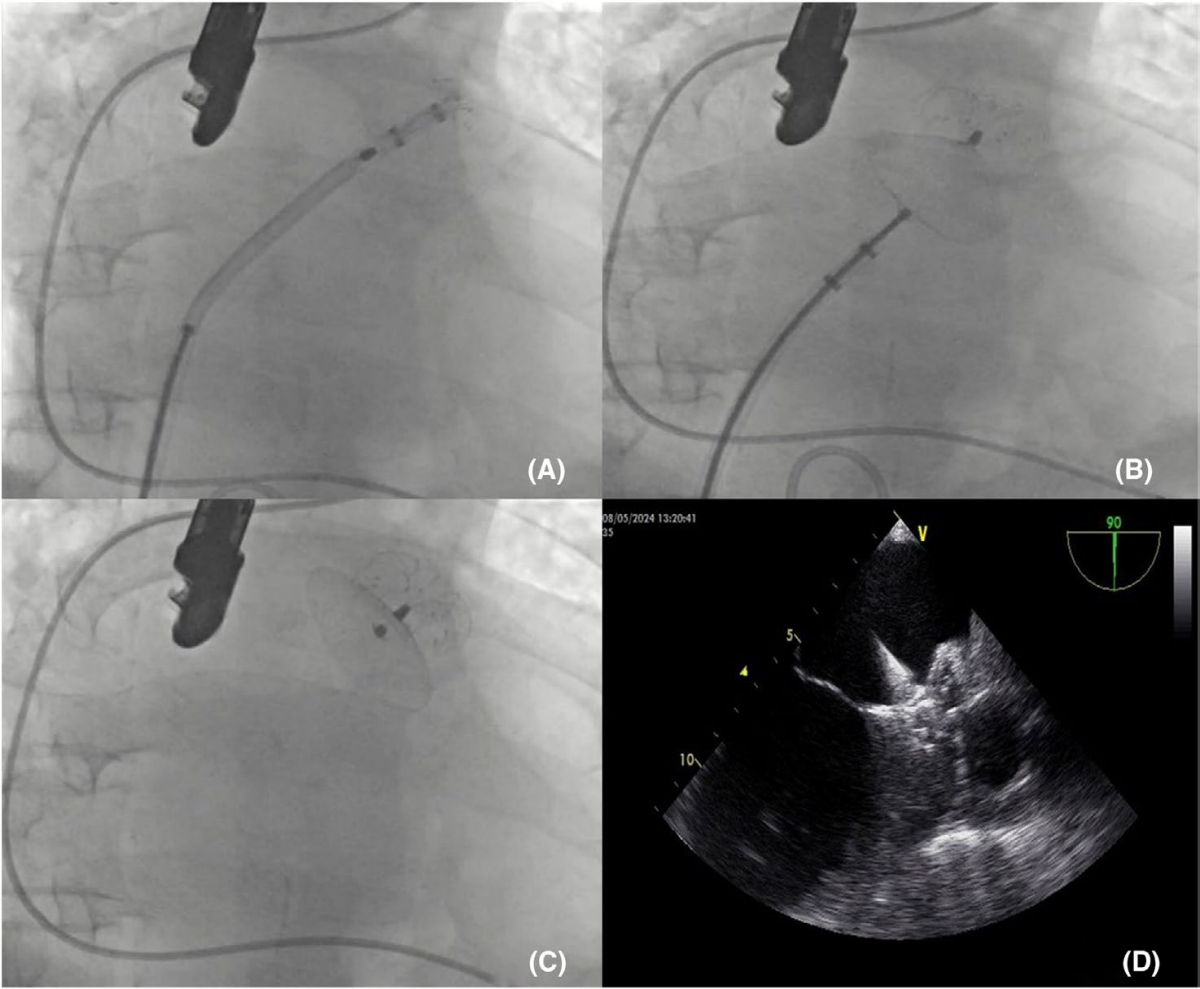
Left Atrial Appendage Closure (LAAC) with the LAmbre™ Device in Case 2. (A) The delivery sheath demonstrates the ball‐shaped LAmbre™ device. (B) Tug test confirming the stability of the device before release. (C) Final release of the device. (D) Intraoperative transesophageal echocardiography (TEE) image postdevice deployment showing proper positioning and sealing.
